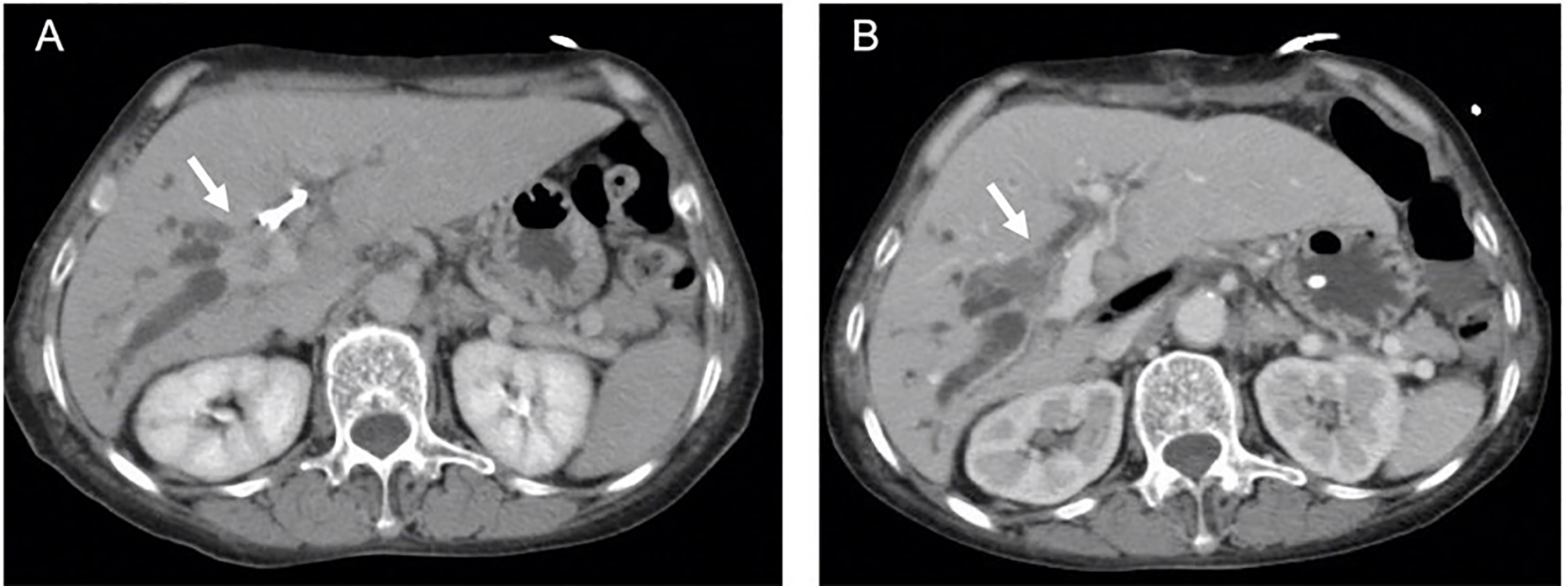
(A) Arrow indicates enhanced Bismuth type IIIA perihilar cholangiocarcinoma (PHCC) with percutaneous biliary drainage (PTBD) tube in computed tomography (CT) scan. (B) No evidence of enhanced tumor (arrow) with patent biliary tract was observed in CT scan at one month after irreversible electroporation treatment (IRE).
